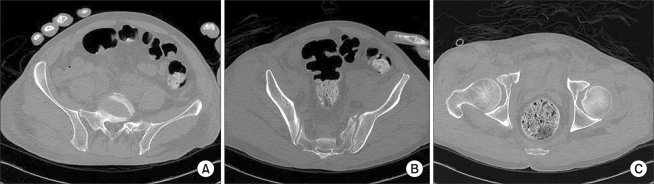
Pelvis computed tomography showed extra-articular fracture of the right acetabulum (A), sacral alar (B), and anterior portion of the sacroiliac joint on the left (C).
